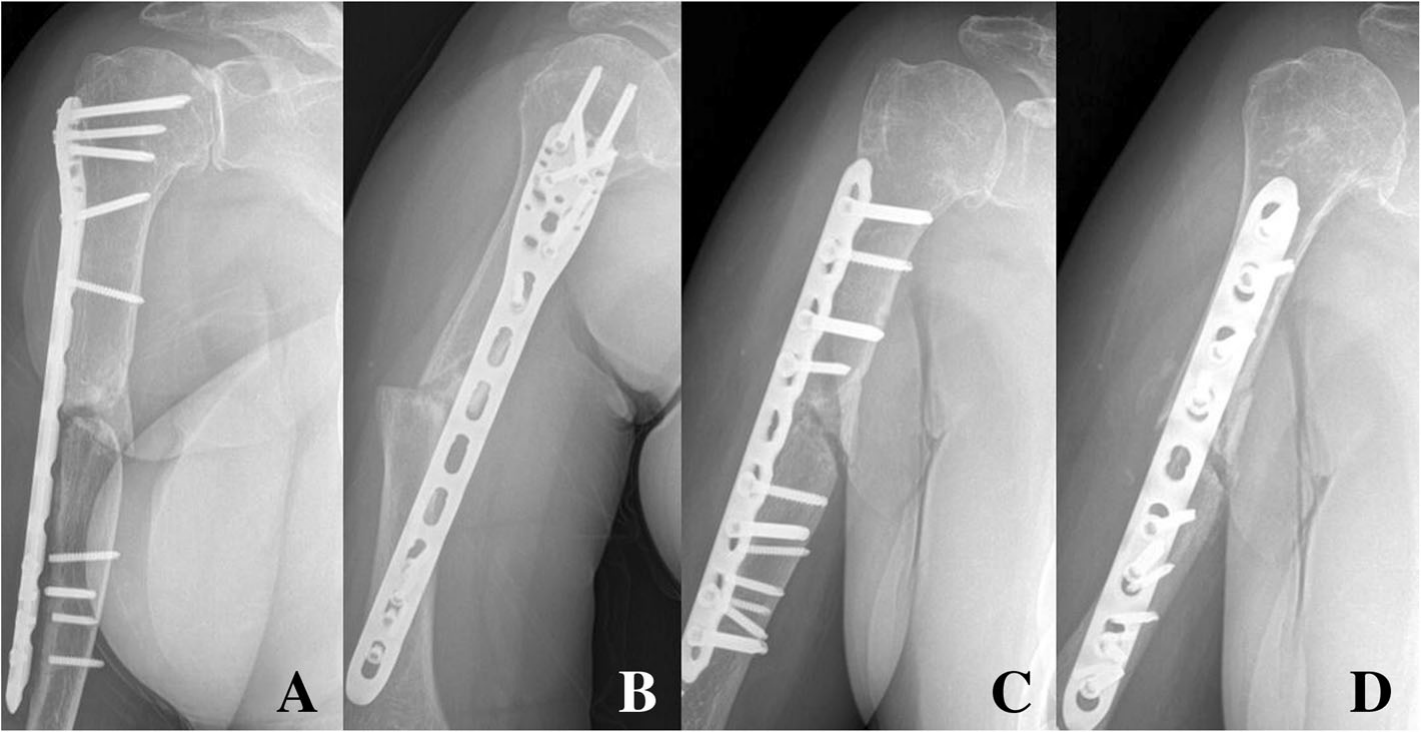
Anteroposterior and lateral X-ray showing the metal failure of spiral (Spiral wedge type, AO classification: B1) humerus shaft fracture of in a 74-year-old female patient (a, b). Using open approach, narrow locking compression plate was used with bone graft (c, d)
